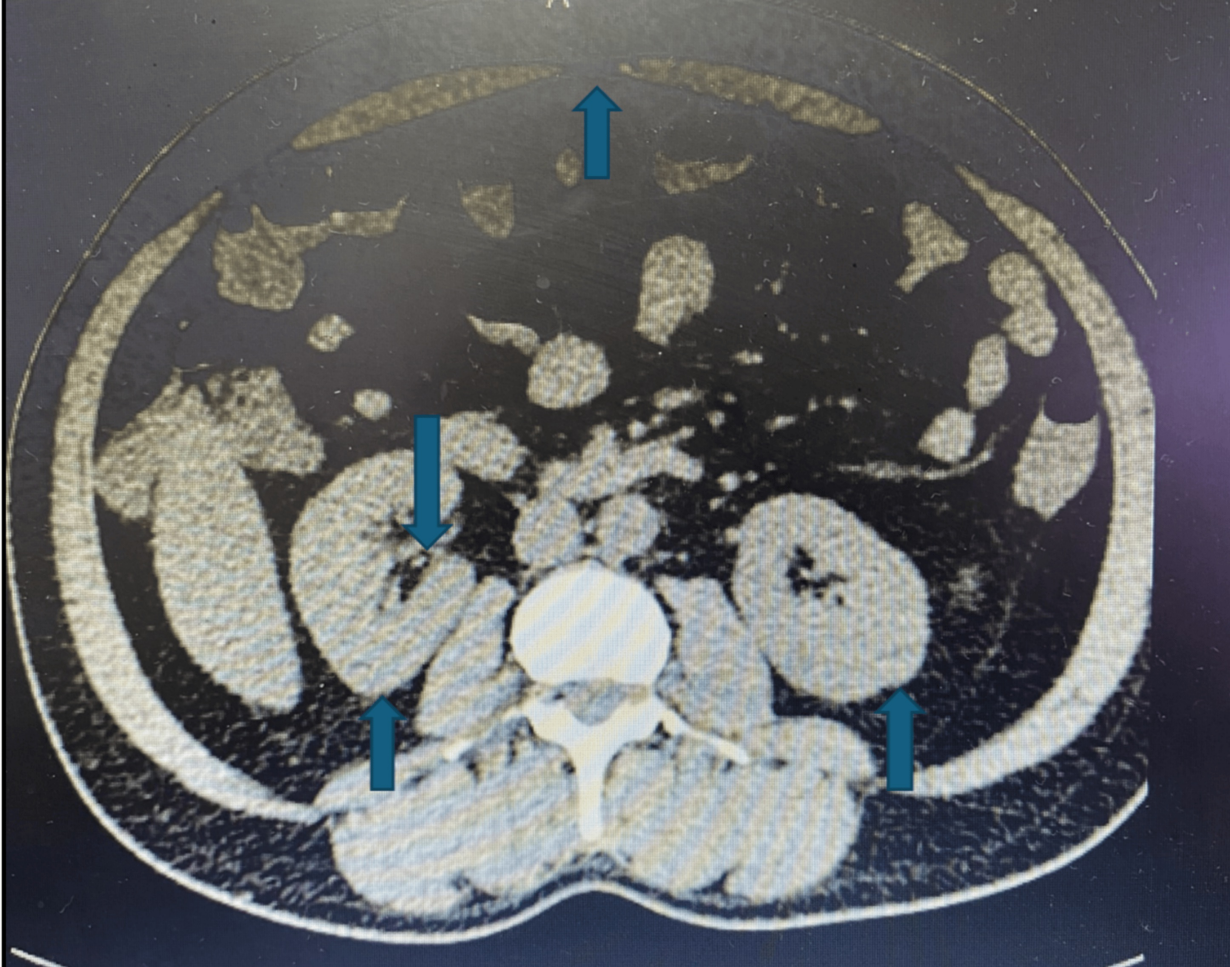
Computed tomography of the whole abdomen showing tiny right nephrolithiasis in the lower pole of the calyx, bilateral simple renal cortical cysts-Bosniak category 1, and small umbilical hernia.
